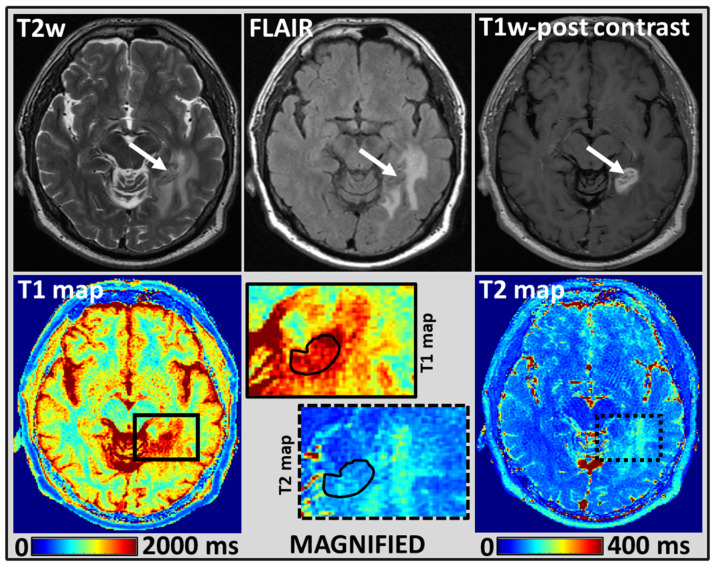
Representative images from a 59-year-old female patient treated with focal RT and had a history of primary lung cancer: T2w (arrow pointing to the lesion), T2w FLAIR, and T1w post-contrast images from the clinical scan, which demonstrate a left temporal lobe enhancing metastasis (top row). A black solid and dotted rectangle region on T1 and T2 maps, respectively, were magnified, and the placement of a manually drawn ROI for the BM lesion was shown in a solid black line. MRF-derived quantitative T1 and T2 maps (bottom row). The T1 and T2 values from the treated BM lesion were 1906 ms and 106 ms, respectively.
